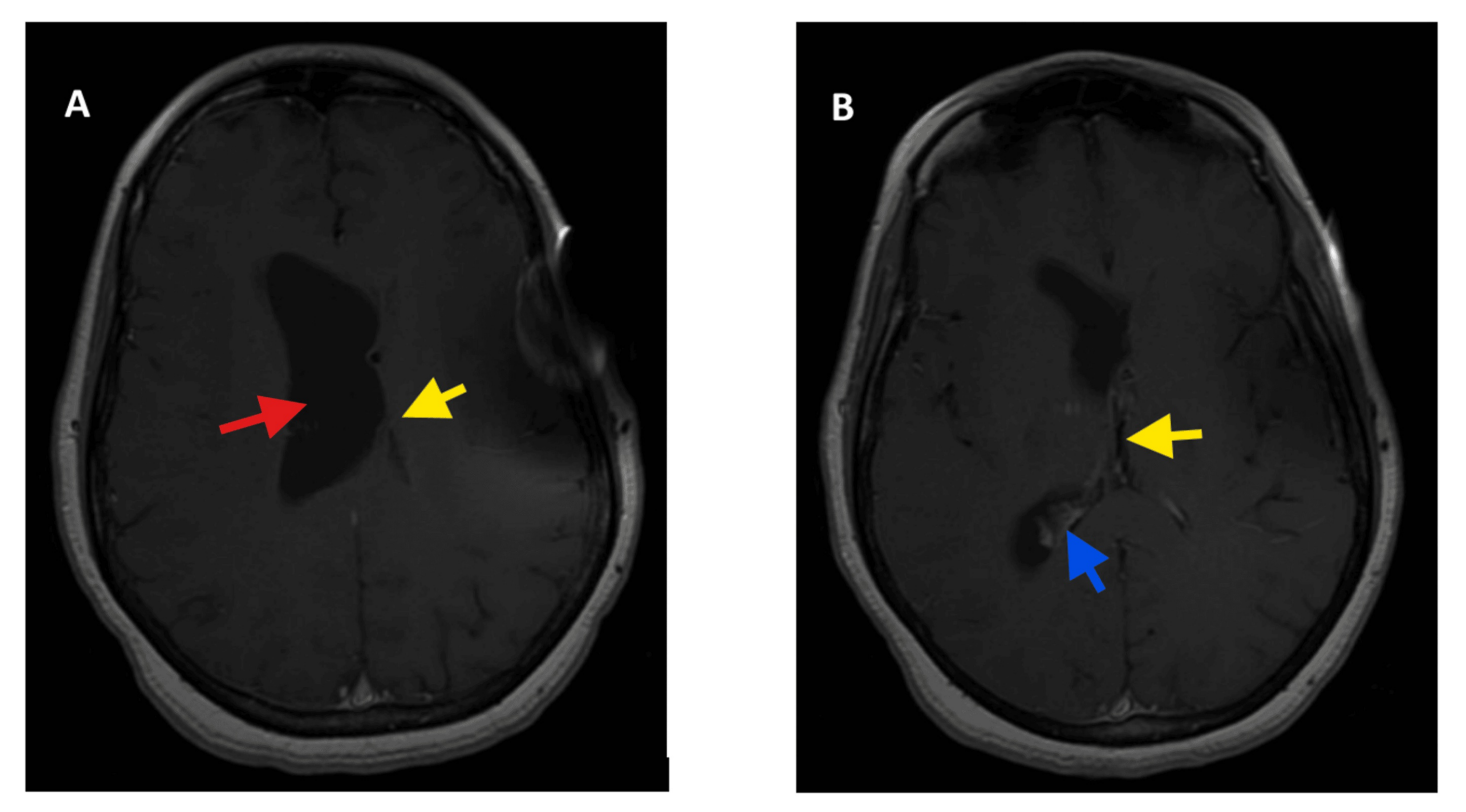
Repeat MRI brain with and without contrast showing (A) stable asymmetric ventriculomegaly involving the right lateral ventricle (red arrow) and (B) significantly decreased debris in the right ventricle (blue arrow). The yellow arrows highlight a decompressed left ventricle
